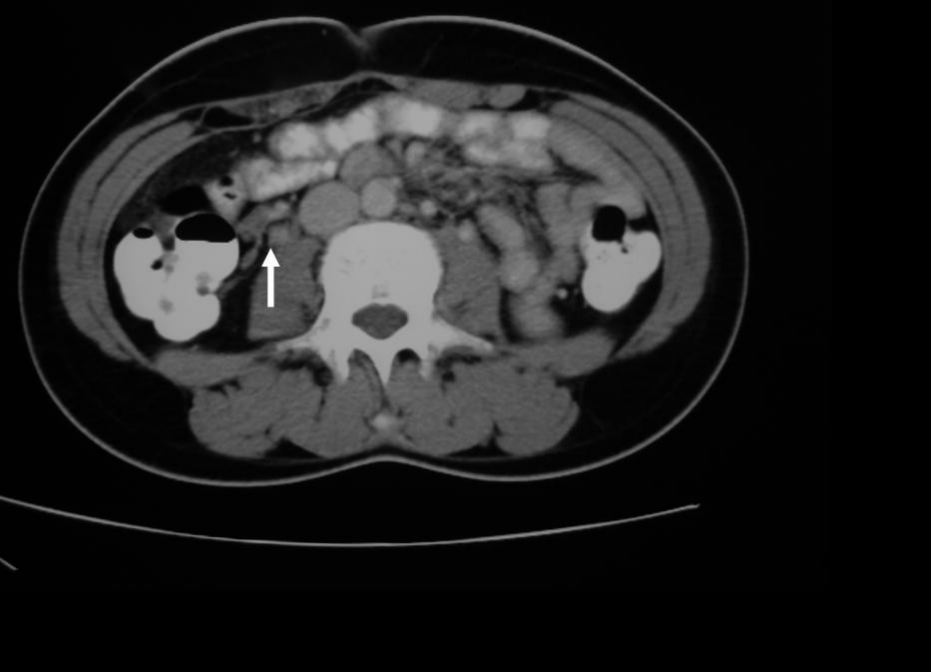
Follow up abdominal CT scan one month after discharge-arrow indicating a patent right ovarian vein.
